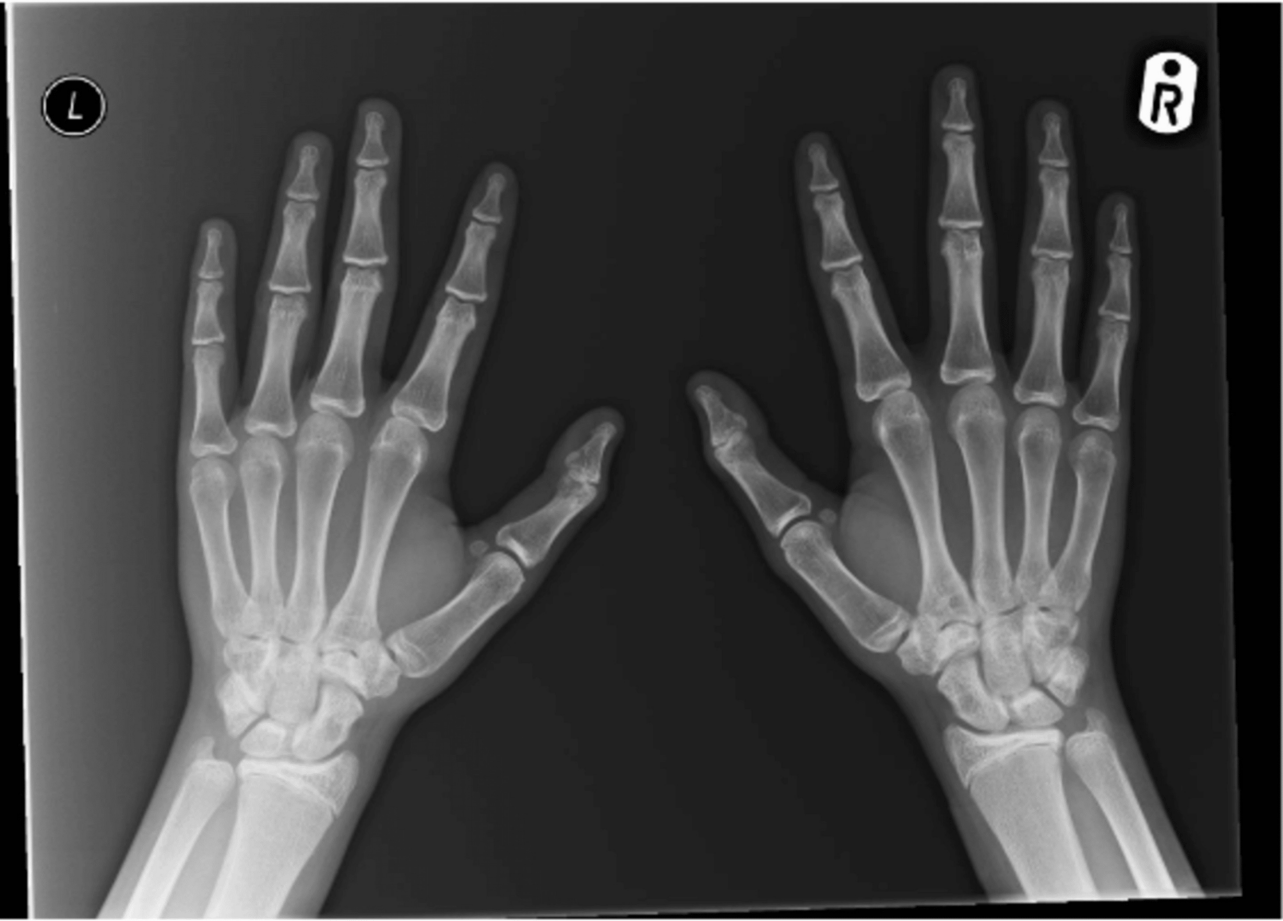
X-ray of the hands with no demonstrated shortening of the metacarpals, often seen in PHP. PHP: pseudohypoparathyroidism
